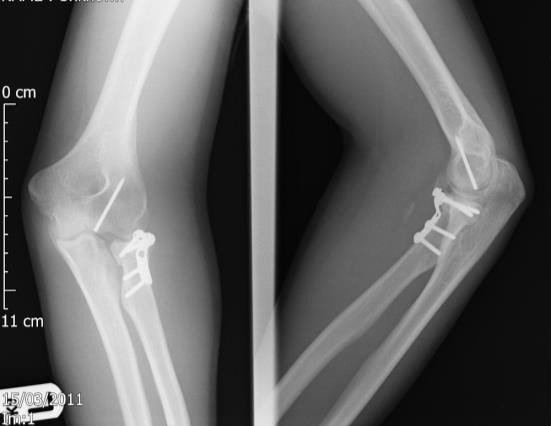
Post-operative plain radiographs after reconstruction and internal fixation of type IV radial head fracture.
